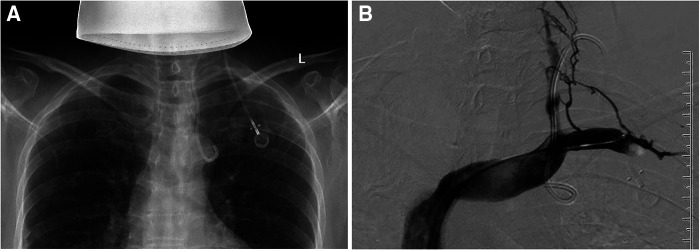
Catheter malposition to an undefined collateral vein. (A) Chest *x*-ray shows that the tip of the catheter is located at the aortic arch; (B) Fluoroscopy showed that the tip of the catheter turns back into an angle under the left clavicle, and DSA radiography shows that the vein where the catheter is located is not visible, considering that the head end enters the venule branch.
